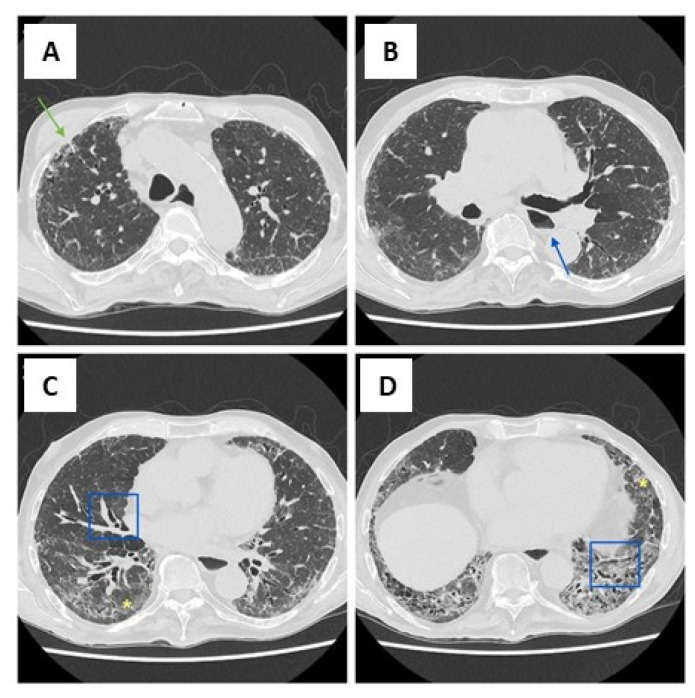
Radiological images of a 72-year-old man with SSC-ILD (A–D). CT scans show the main features of NSIP, which is the most common pattern in patients with systemic sclerosis. In particular, traction bronchiectasis (in the blue square) (C,D), ground glass areas (yellow asterisk) (C,D) with a symmetrical distribution and mainly peripheral and lower lobe involvement, and reticular opacities (green arrow) (A) are noted. The blue arrow also indicates the dilated esophagus (B), another feature observed in patients with systemic sclerosis.
